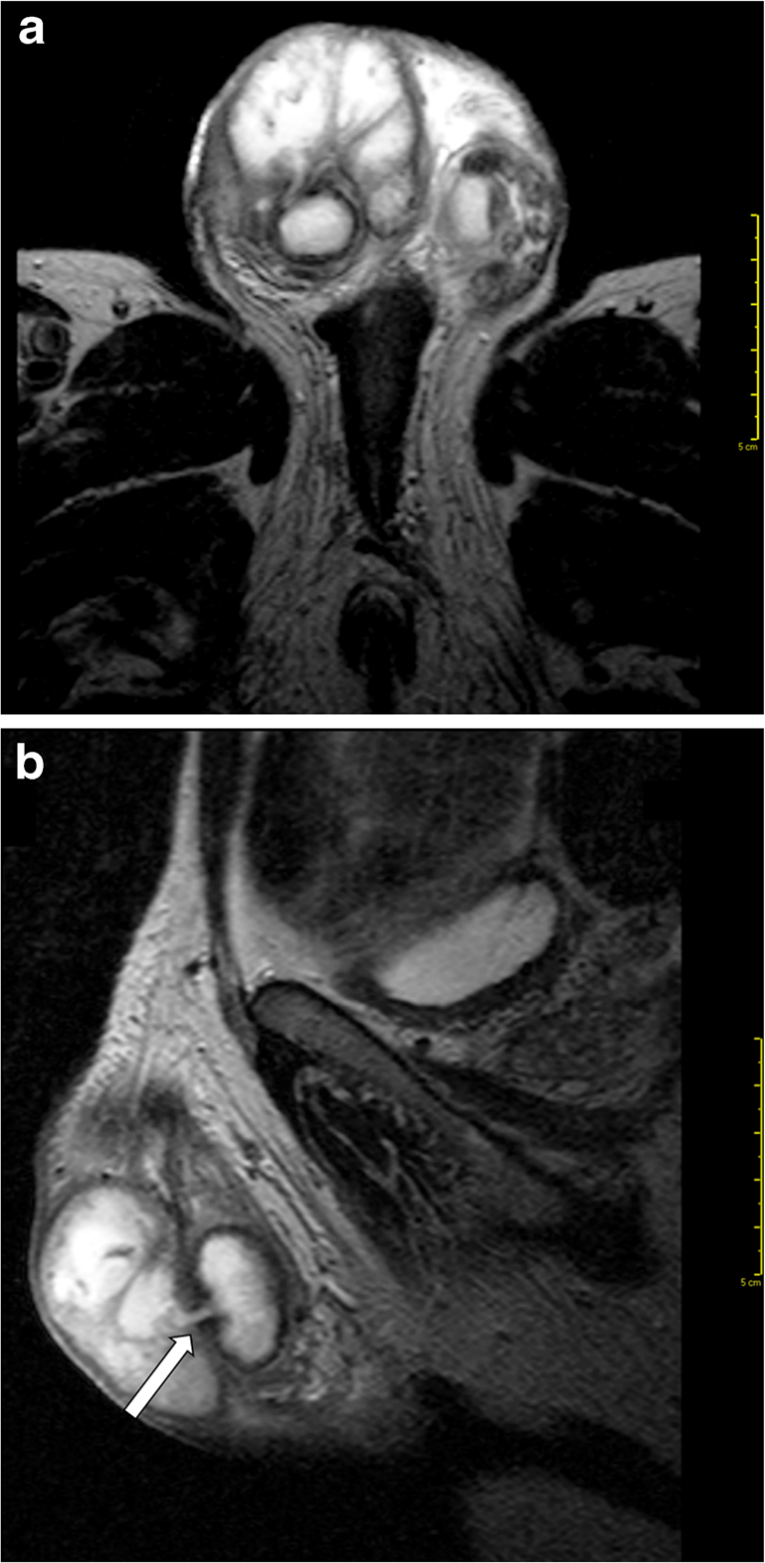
a-b Intrascrotal abscess. a T2-weighted TSE sequence, axial view, and b T2-weighted TSE sequence, sagittal view, showing fistulous track (arrow)
